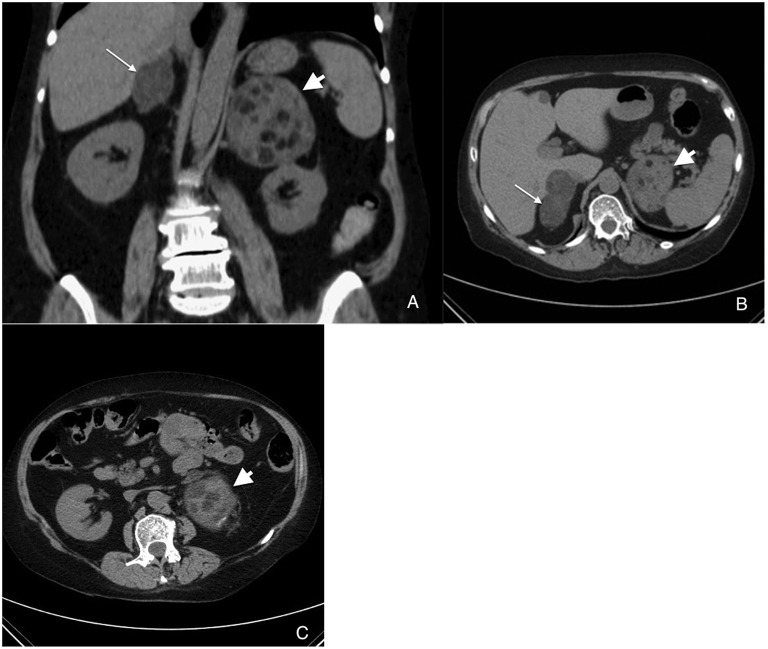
Coronal (A) and axial (B,C) views of adrenal CT scan showing bilateral adrenal enlargement with features of mixed BMAH (right; thin arrow) and myelolipoma (particularly on left; short arrow).
